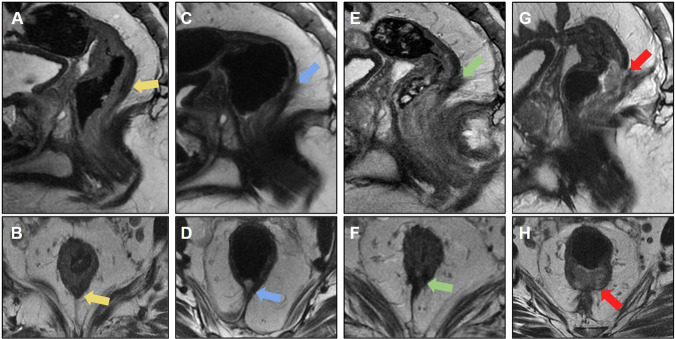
A Sagittal and B axial T2-weighted images. A patient with distal rectal cancer underwent local resection (pT2). MRI revealed a low signal intensity scar (yellow arrow) with no evidence of mesorectal disease. C, D Follow-up MRI at 5 months revealed missed changes within the mesorectal fat posterior to the scar (blue arrow). E, F Follow-up MRI at 1 year demonstrating missed scar recurrence extending along the mesorectal fat (green arrow). Changes from fat stranding to a nodular lesion along the mesorectal fat near the scar should raise suspicion of recurrence after local resection. Endoscopic studies failed to detect extraluminal recurrence. G, H Posterior follow-up at 1 year and 6 months revealed a mucosal lesion (red arrow). Local recurrence was detected using endoscopy with a positive mesorectal fascia. Treatment with chemoradiotherapy and total mesorectal excision was indicated
